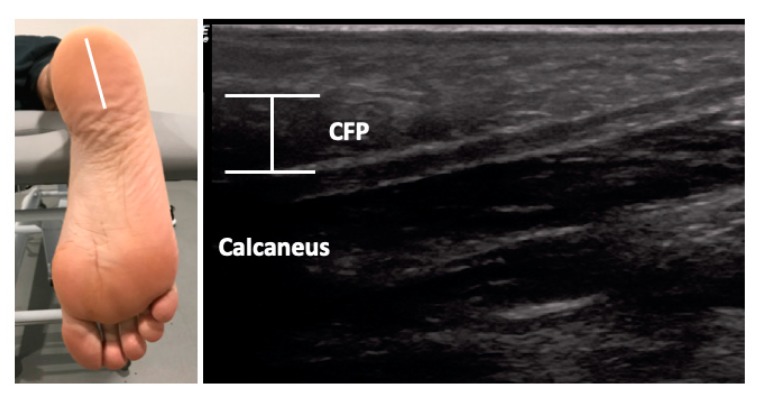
Ultrasound imaging thickness for the calcaneal fat pad in an individual with AT. Abbreviations: CFP, calcaneal fat pad.
